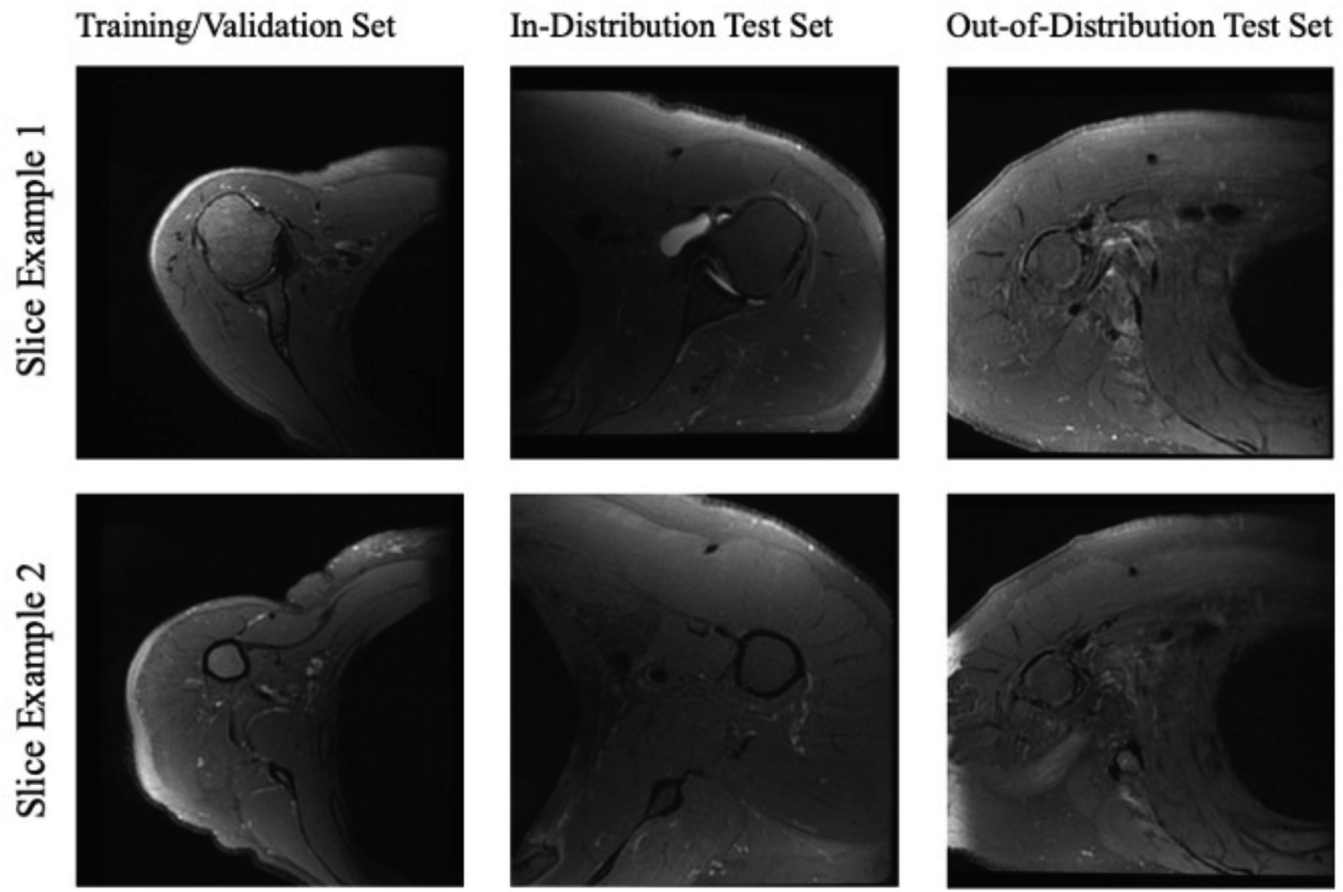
Example slices of MRIs used in this study. *MRI*, magnetic resonance imaging.
